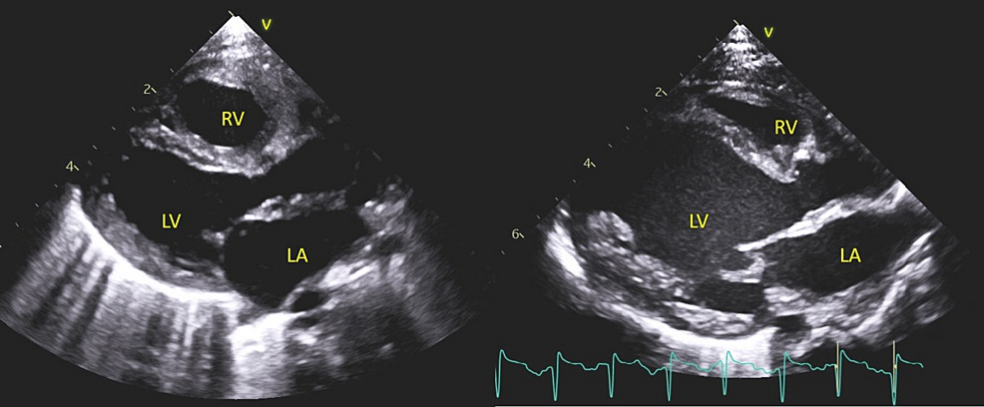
Parasternal long axis view showing the difference in LV size (left of screen done at DOL 2 – normal size) (right of the screen done at DOL 20 – severely dilated LV) DOL: Days of life
